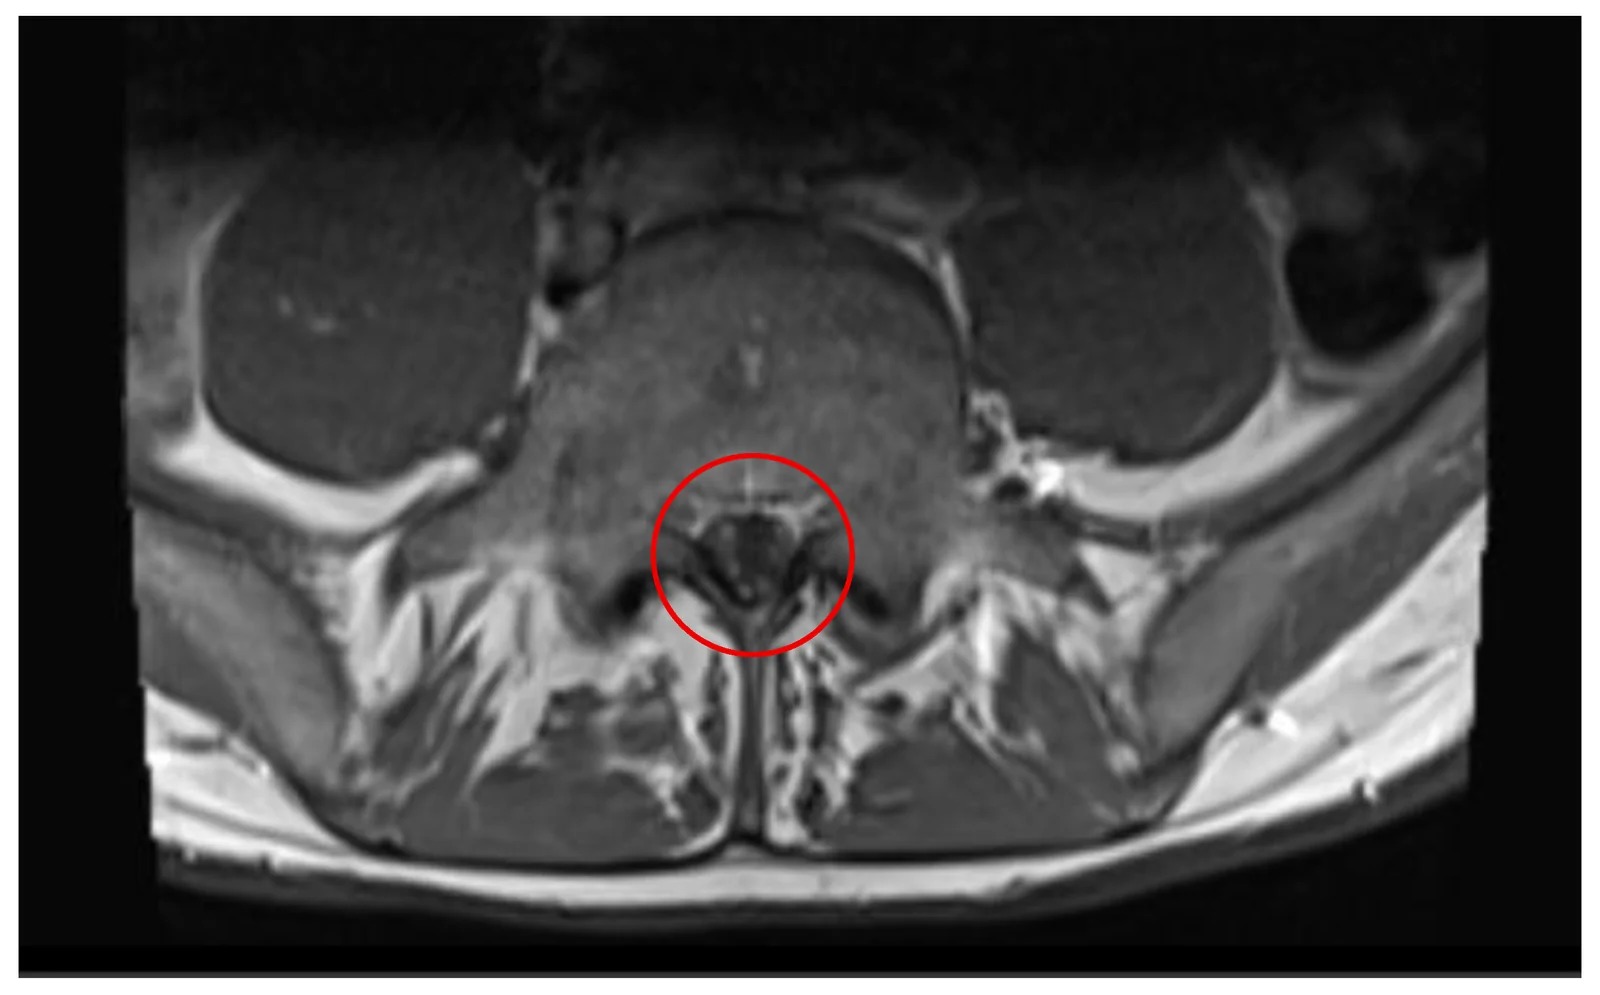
Axial contrast-enhanced T1-weighted spinal MRI showing enhancement of the cauda equina nerve roots (encircled).
